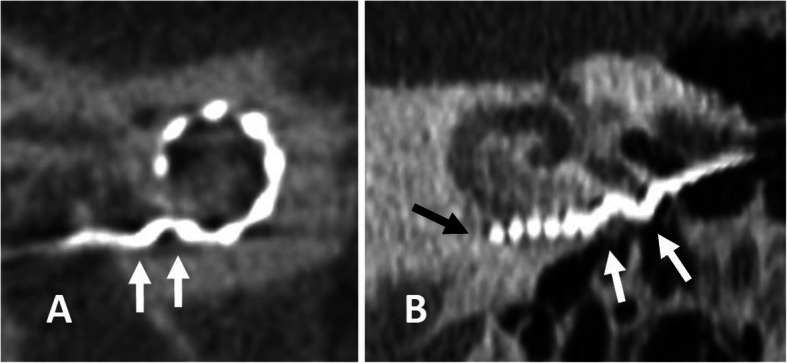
a A 75-year-old female patient, with electrode bending. CBCT paraaxial image shows electrode bending (arrows) at the basal turn. b A 70-year-old male patient with electrode pinching in far advanced otosclerosis. CBCT paraaxial image shows accordion-like pinching of the basal parts of the electrode array (white arrows). The tip of the electrode array sticks at the basal turn and does not turn around the modiolus (black arrow)
